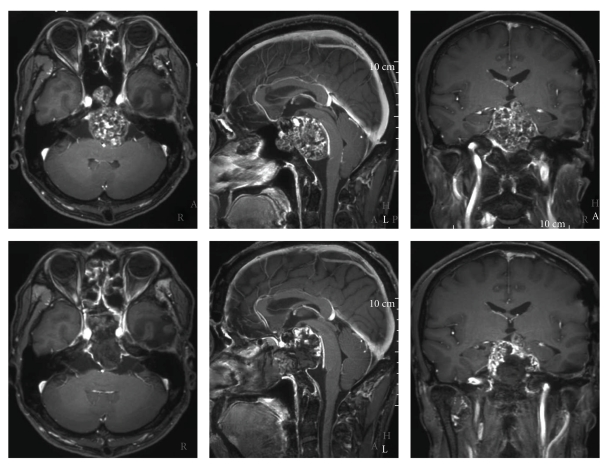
Case 2. Upper: MRI images after the second operation. Lower: MRI after transsphenoidal surgery. Tumor was resected except suprasellar part and decompressed brainstem.
